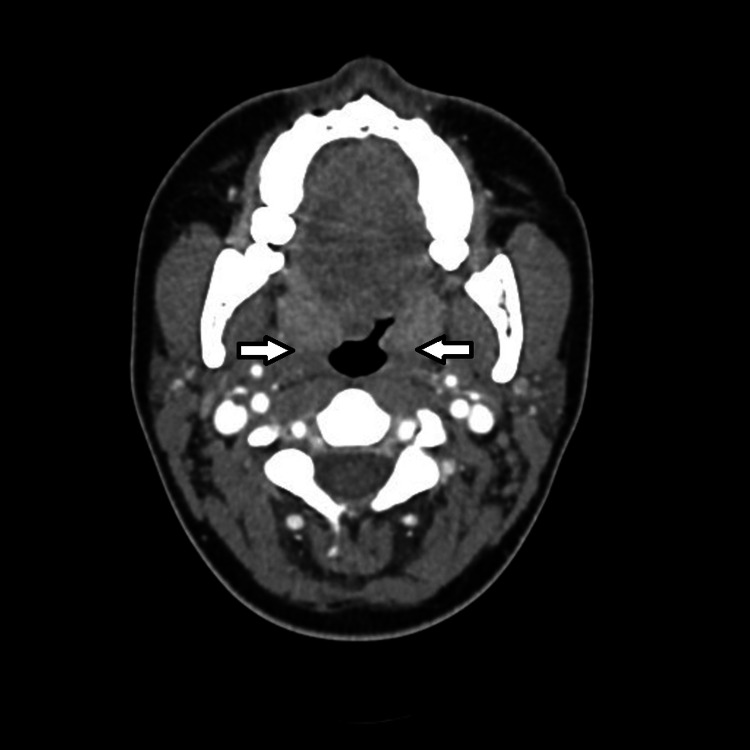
Initial CT with contrast demonstrating bilateral palatine tonsil enlargement.
